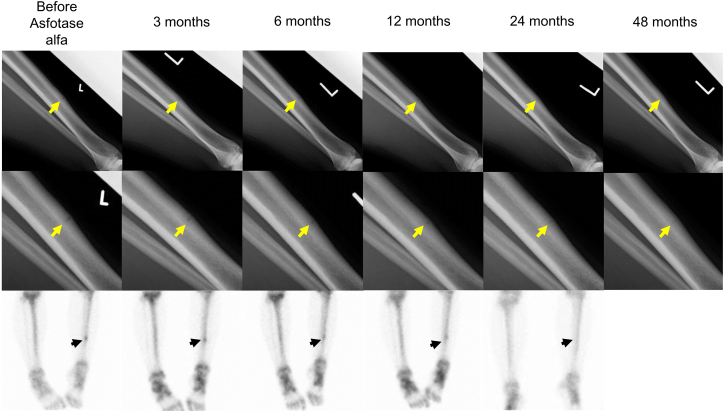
Radiographic improvement of a pseudofracture in the patient with HPP following asfotase alfa treatment (upper line: X-rays, middle line: enlarged images of X-rays, lower line: bone scintigraphy). Improvement of pseudofracture was detected by both X-ray (yellow arrows) and bone scintigraphy (black arrows). In the X-ray images, the radiolucent band became obscured but remained at one year and finally disappeared two years after the initiation of asfotase alfa. By bone scintigraphy, radionuclide accumulation began to diffuse at six months and disappeared as early as 24 months.
